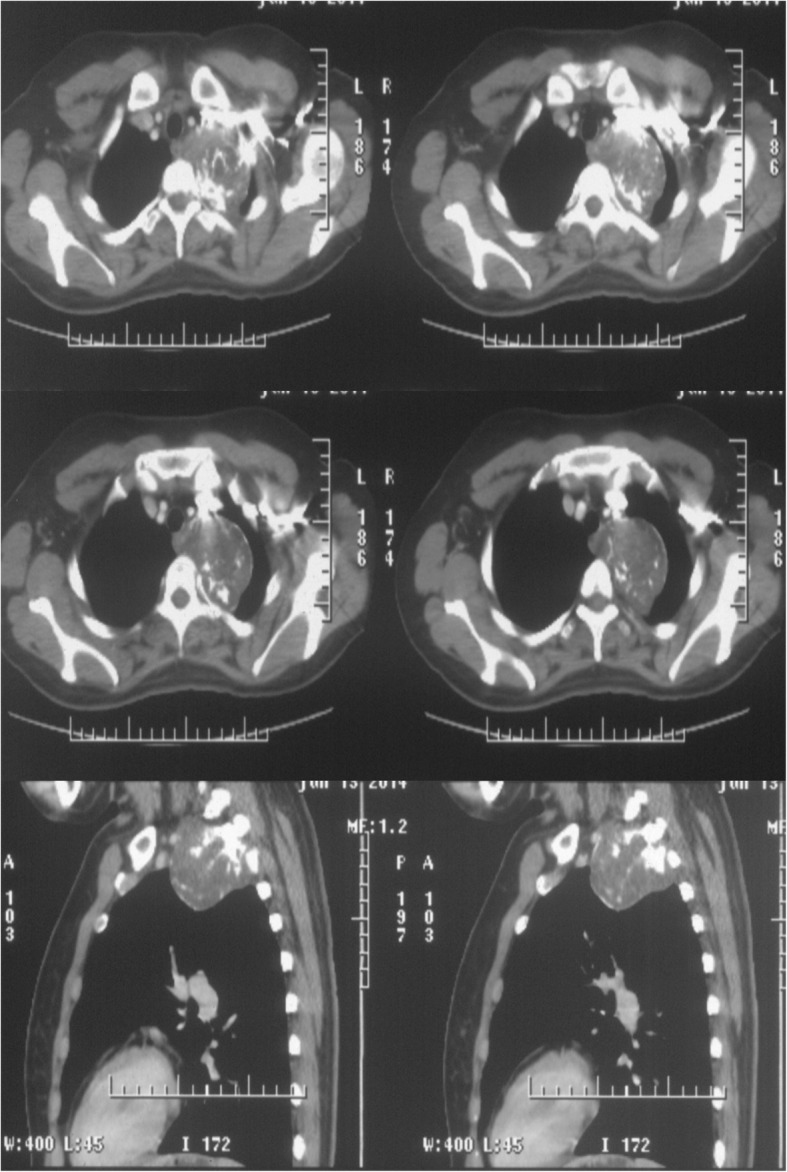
The contrast-enhanced CT scans of the chest. There was a round, soft tissue density mass in the posterior superior mediastinum. The mass with high-density shadow was related to the left second rib in which soup bubbly appearance and expansion can be found
